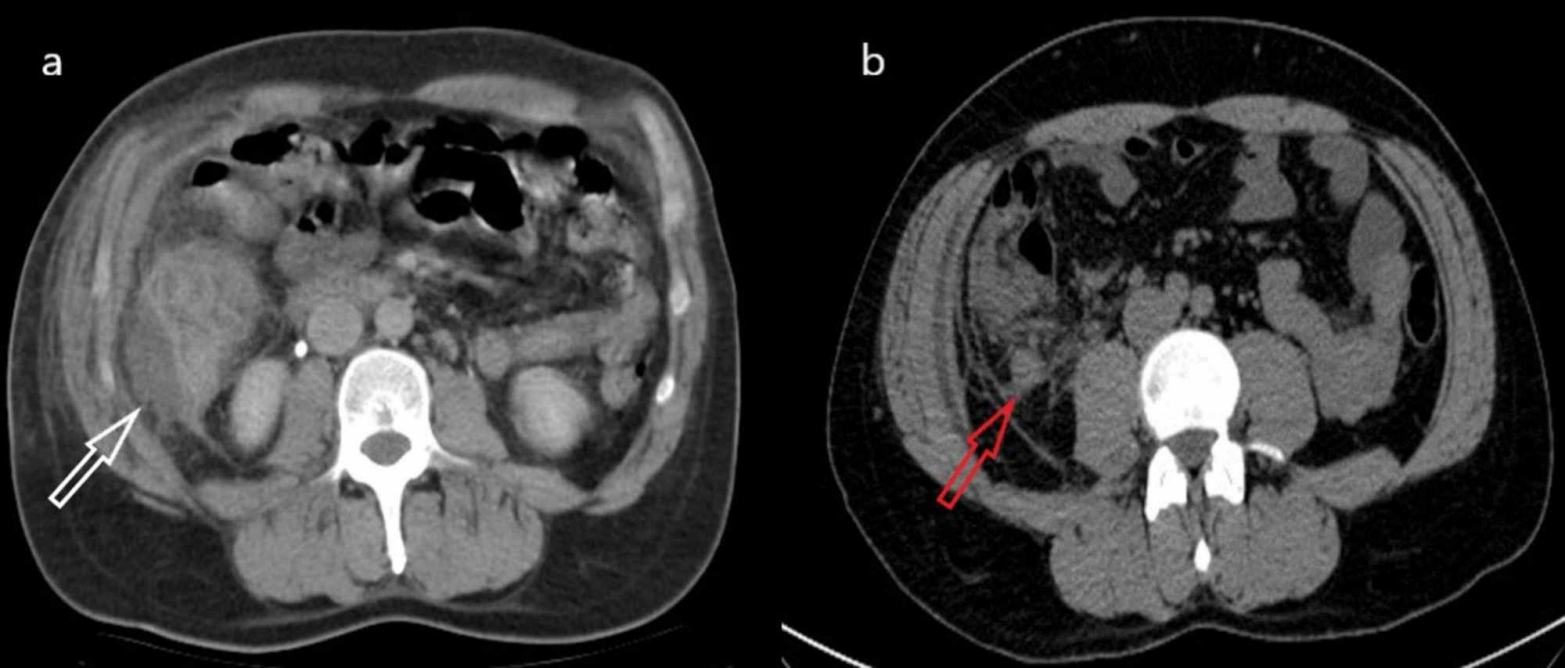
a) Axial non-contrasted abdominal CT scan, showing a collection adjacent to the ascending colon signaled by the white arrow; notice the surrounding stranded fat. b) Axial image of the same CT scan, with the red arrow showing an enlarged appendix with surrounding inflamed fat.
